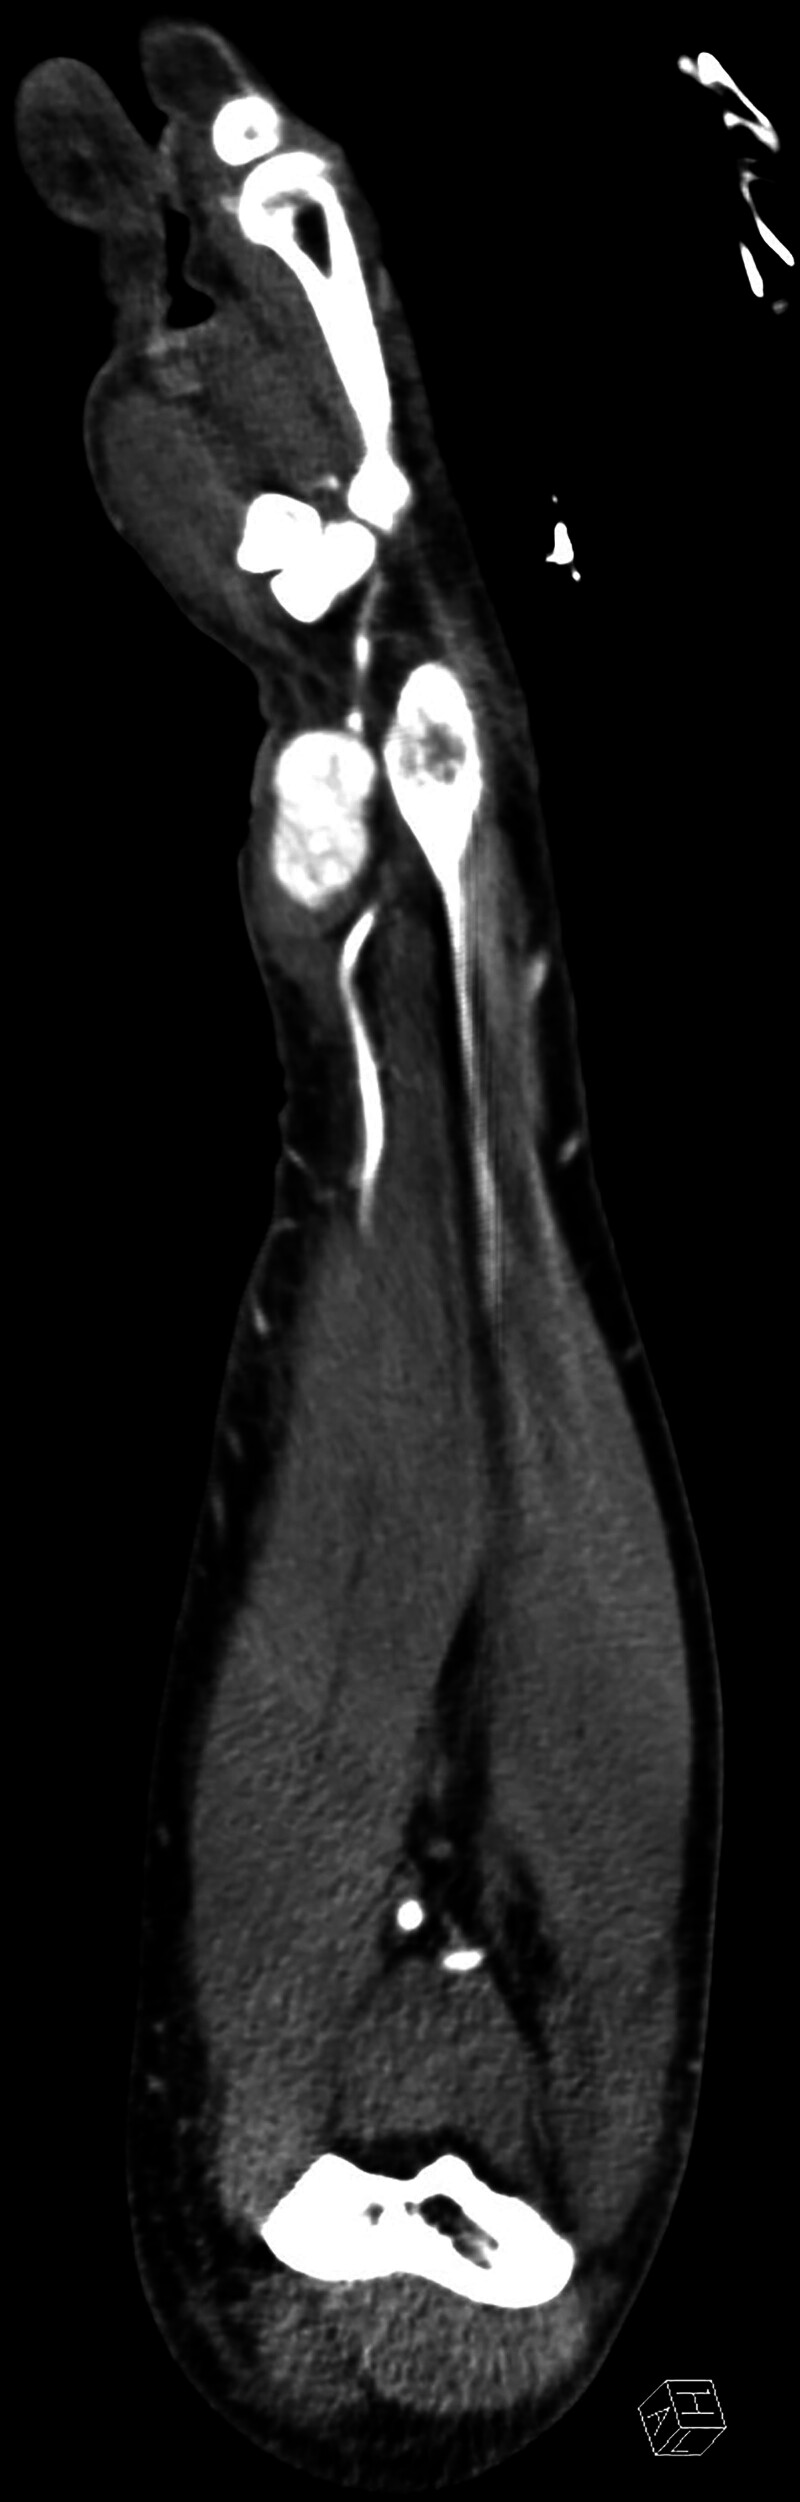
Computed tomography angiography revealing a pseudoaneurysm of the radial artery as the underlying cause of median nerve compression by the resulting large hematoma.
